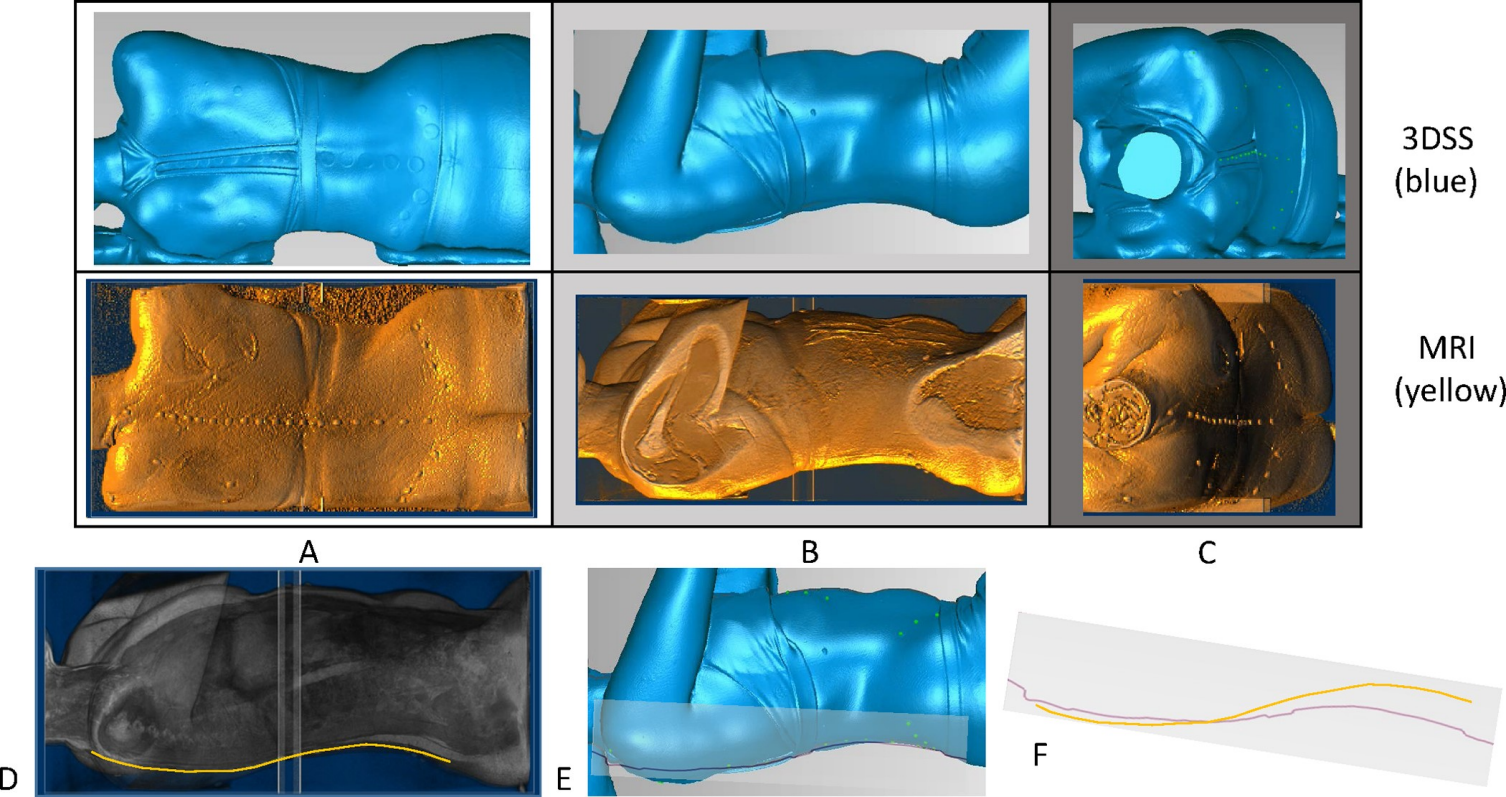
Posterior, Right lateral and Superior view of the skin envelope over the torso. Posterior (A), Right lateral (B) and Superior (C) view of the skin envelope over the torso for participant 8, reconstructed from 3DSS (blue) and MRI (yellow) data. The difference in participant position, body shape and shoulder/hip orientation is evident in all three orientations, and highlighted by comparing the trajectory of the fiducial surface markers on the spinal column in both A and C. Comparing a line demarcating the sagittal profile of surface markers on the MRI (D, yellow) and 3DSS (E, purple) it is evident that these profiles differ both in shape and curvature (F).
